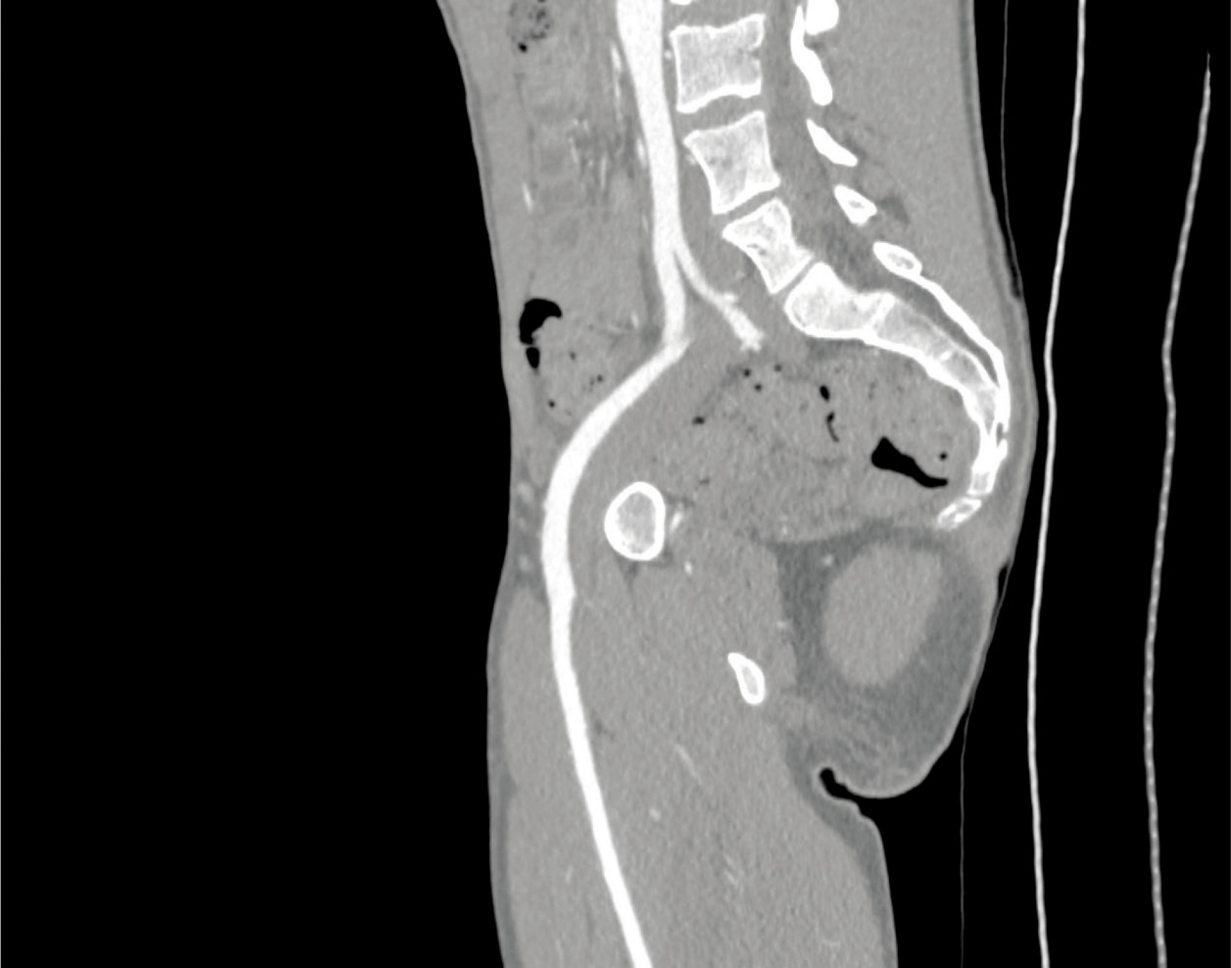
A reconstructed sagittal computed tomographic angiogram of the abdominal aorta and the left iliac arteries using the stretch reformation technique, revealing a stenosis and kink in the proximal half of the left external iliac artery (an endofibrotic plaque). Poststenotic dilation is noted. (The patient agreed to the publishing of his radiological images.)
